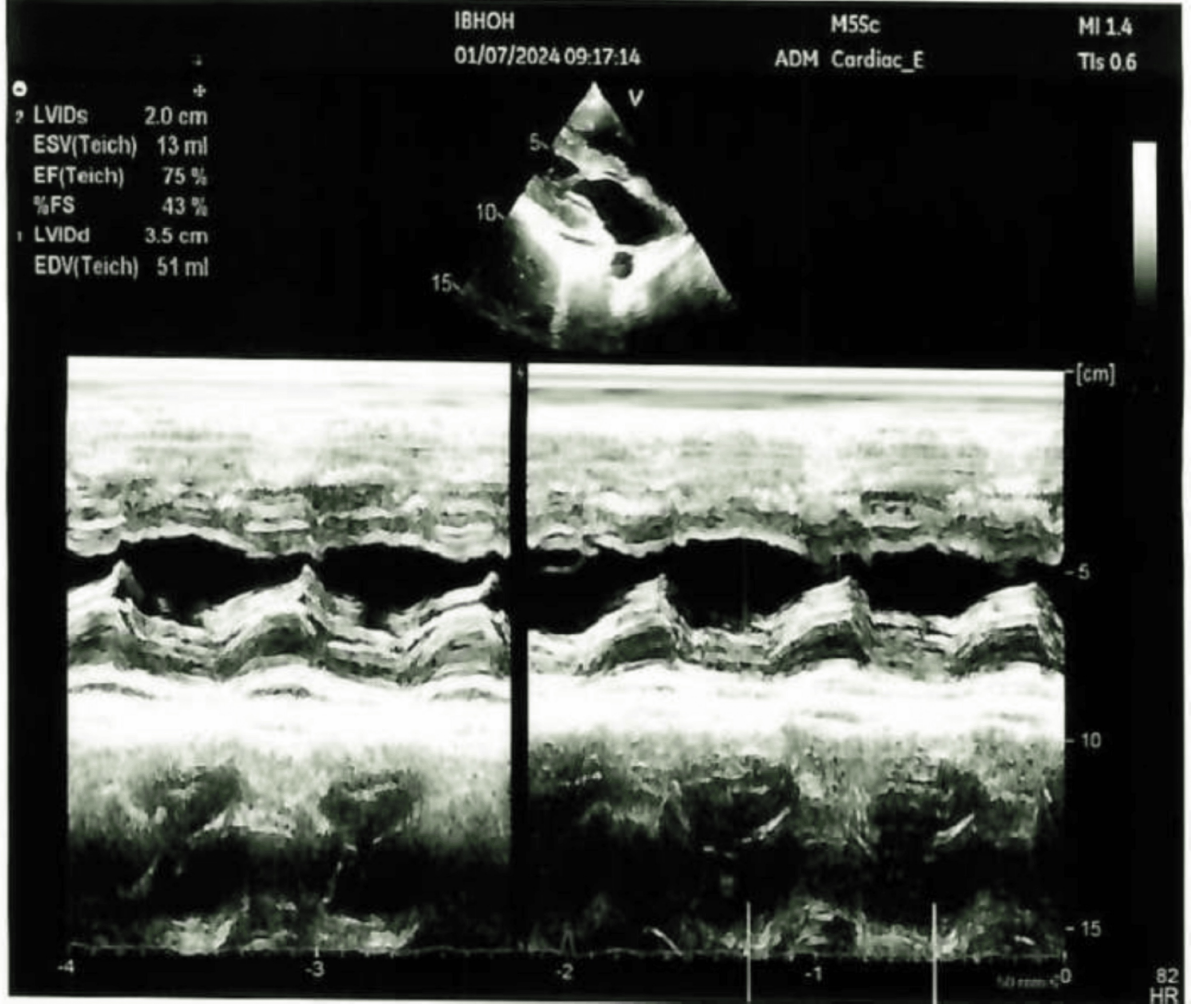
Echocardiography No abnormality detected.
